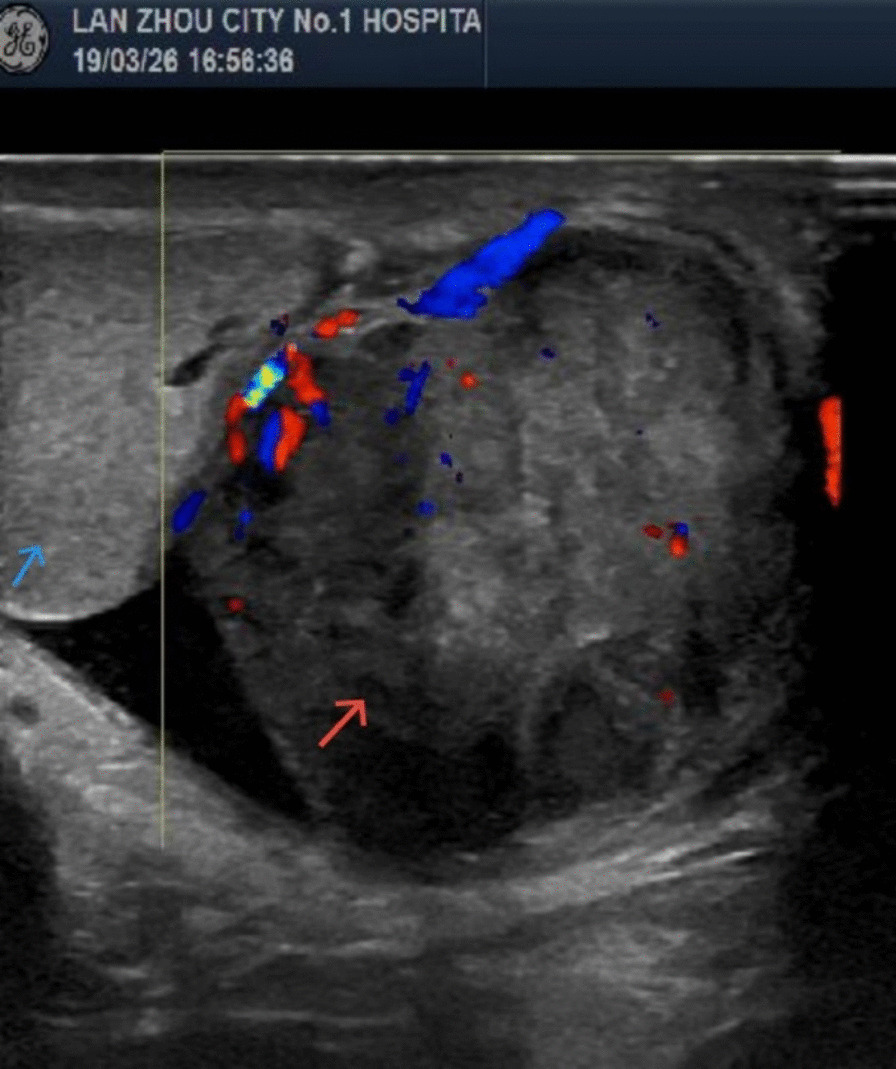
Scrotal ultrasound showing a well-defined hypoechoic paratesticular mass (red arrow) located in the inferior aspect of the right testicle (blue arrow), measuring approximately 4.5 cm × 3.5 cm × 4 cm
